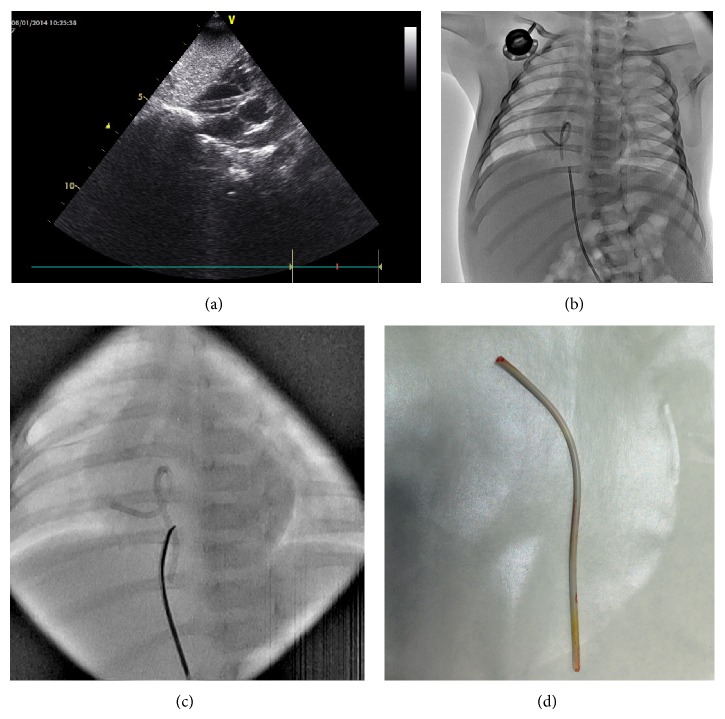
(a) Echocardiographic and (b) angiographic images of an embolized catheter making a loop in the heart of a 4.5-month-old infant and (c) images of its retrieval via snare are shown. (d) The embolized port catheters.
